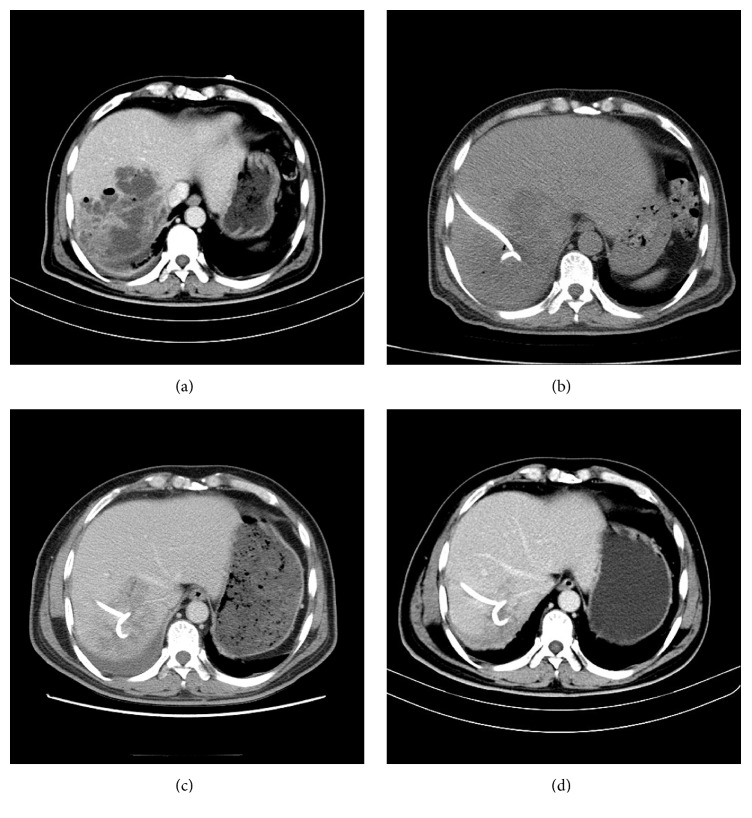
A 43-year-old male presented with fever and right abdominal pain for five days. (a) Enhanced CT shows a lager multiloculated liver abscess in the right hepatic lobe. The abscess cavity has gas formation, which is associated with mortality. (b) Catheter was placed under CT guidance. (c) After the pus was pulled out, oxygen-ozone gas mixture was given through catheter and filled the small locules of the abscesses; some separation was broken. (d) Abscess was absorbed after three weeks.
